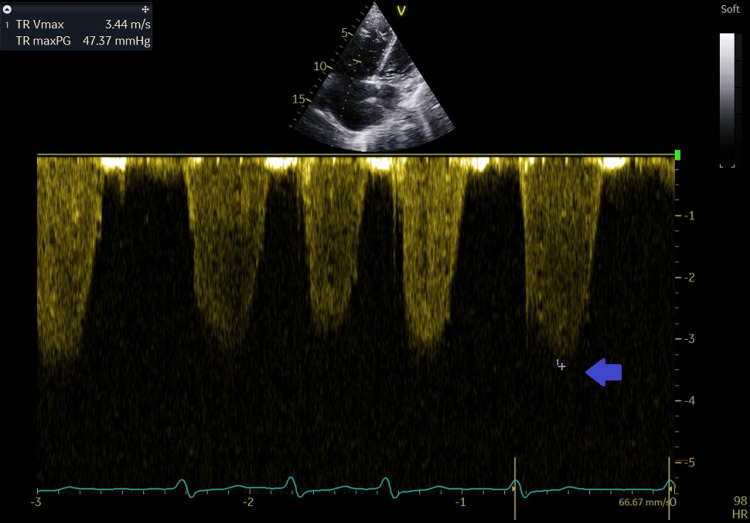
Tricuspid regurgitation jet velocity recorded with continuous wave Doppler at 3.4 m/second (blue arrow).
